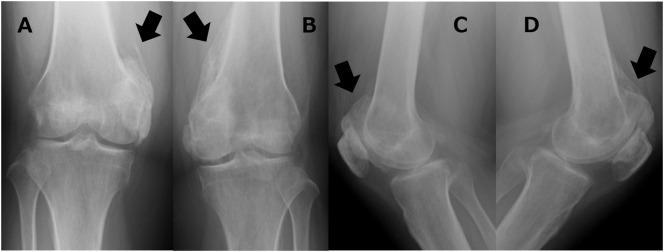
Preoperative anteroposterior and lateral radiographs of the knees showing heterotopic ossification on the peripheral areas (arrow). A: anteroposterior radiographs of the right knees B: anteroposterior radiographs of the left knees C: lateral radiographs of the right knees D: lateral radiographs of the left knees.
